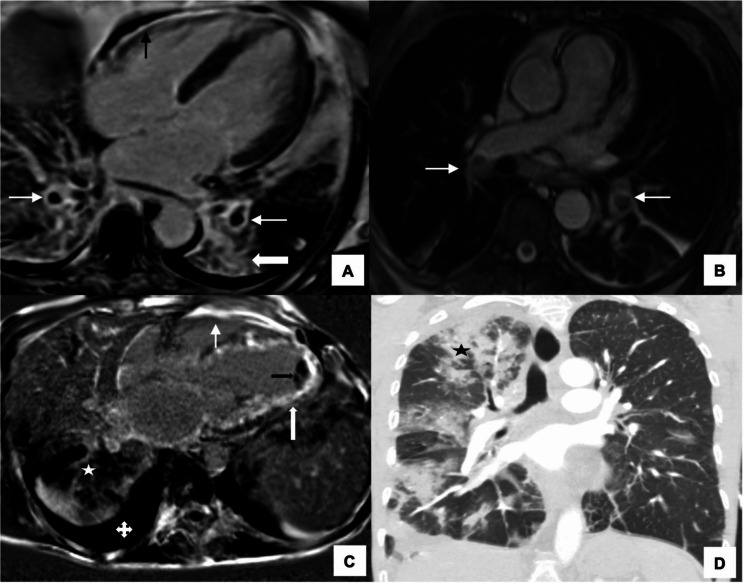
Lung disease associated with cardiac EGPA. A-B: Pulmonary embolism and pericarditis. A: CMR 4 chamber view shows bilateral segmental PE in both lower lobes (*thin white arrows*) with basal pulmonary infarction (*block arrow*). There is also intense smooth pericardial enhancement in keeping with pericarditis (*black arrow*). B: Axial images show bilateral pulmonary emboli within the lobar right and left lower lobe pulmonary arteries (*arrows*). C-D: Biventricular involvement with pulmonary infiltrates. C: 4 chamber CMR imaging in a 40-year-old with EGPA shows diffuse biventricular LGE (right ventricle, *thin arrow*; left ventricle, *block arrow*) with a LV apical thrombus (*black arrow*). There is ill-defined right lung pulmonary consolidation (*star*) and pleural effusion (*quad arrow*). D: The corresponding CT thorax better delineates the pulmonary parenchymal abnormality (*star*). Abbreviations: CMR, Cardiac magnetic resonance; CT, Computed tomography; EGPA, eosinophilic granulomatosis with polyangiitis; LGE, Late gadolinium enhancement; LV, left ventricle; PE, Pulmonary embolism
